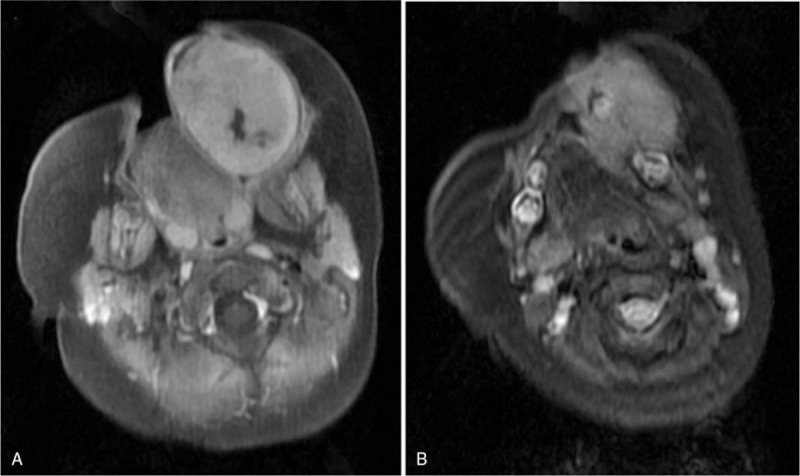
The MRI indicated the presence of a large mass of abnormal signal in the left oral and maxillofacial region that expanded into the peripheral area. The mass was noticeably different from the peripheral tissue on enhanced MRI. The size of the mass was 54 mm × 39 mm × 53 mm. The left maxillary, alveolar bone, and maxillary sinus wall were partly involved.
